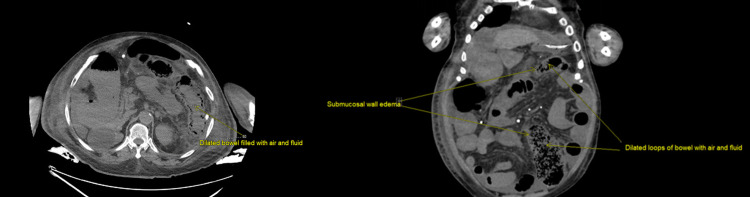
CT scan of the abdomen and pelvis revealing dilated loops of bowel with air and fluid along with submucosal wall edema, findings suggestive of ischemic colitis
